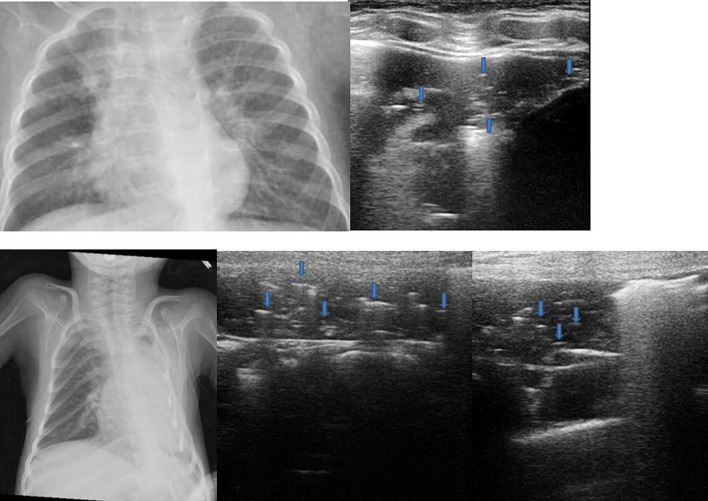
The upper part of the figure shows chest X-ray and lung ultrasound images of a 7-year-old girl with uncontrolled asthma and an ongoing severe asthma attack, with no clinical or laboratory signs of infections. The chest X-ray shows non-specific areas of reduced transparency in the right apical and right basal sites. Grayscale lung ultrasound examination (transverse scan between intercostal fields; linear probe with 12 MHz frequency) shows – on the right paracardiac area – subpleural consolidation with hyperechoic images of air bubbles (static aerial bronchograms, *arrows*) that do not move inward during breathing and are parallel to each other. Their presence is associated with the identification of atelectasis, supported by the clinical and laboratory context of the patient being examined. The lower part of the figure shows chest X-ray and lung ultrasound images of a 4-year-old girl with neuromuscular pathology in nocturnal non-invasive ventilation with ongoing respiratory exacerbation without laboratory and clinical signs of infections. The chest X-ray shows a completely white left lung not well definable. Grayscale lung ultrasound examination (transverse scan between intercostal fields; linear probe with 12 MHz frequency) shows the entire left lung’s subpleural consolidations with hyperechoic images of air bubbles (static aerial bronchograms, *arrows*) static and parallel to each other. Their presence is associated with the identification of left pulmonary atelectasis. The ultrasound suspicion is supported by the clinical and laboratory context of the patient being examined.
